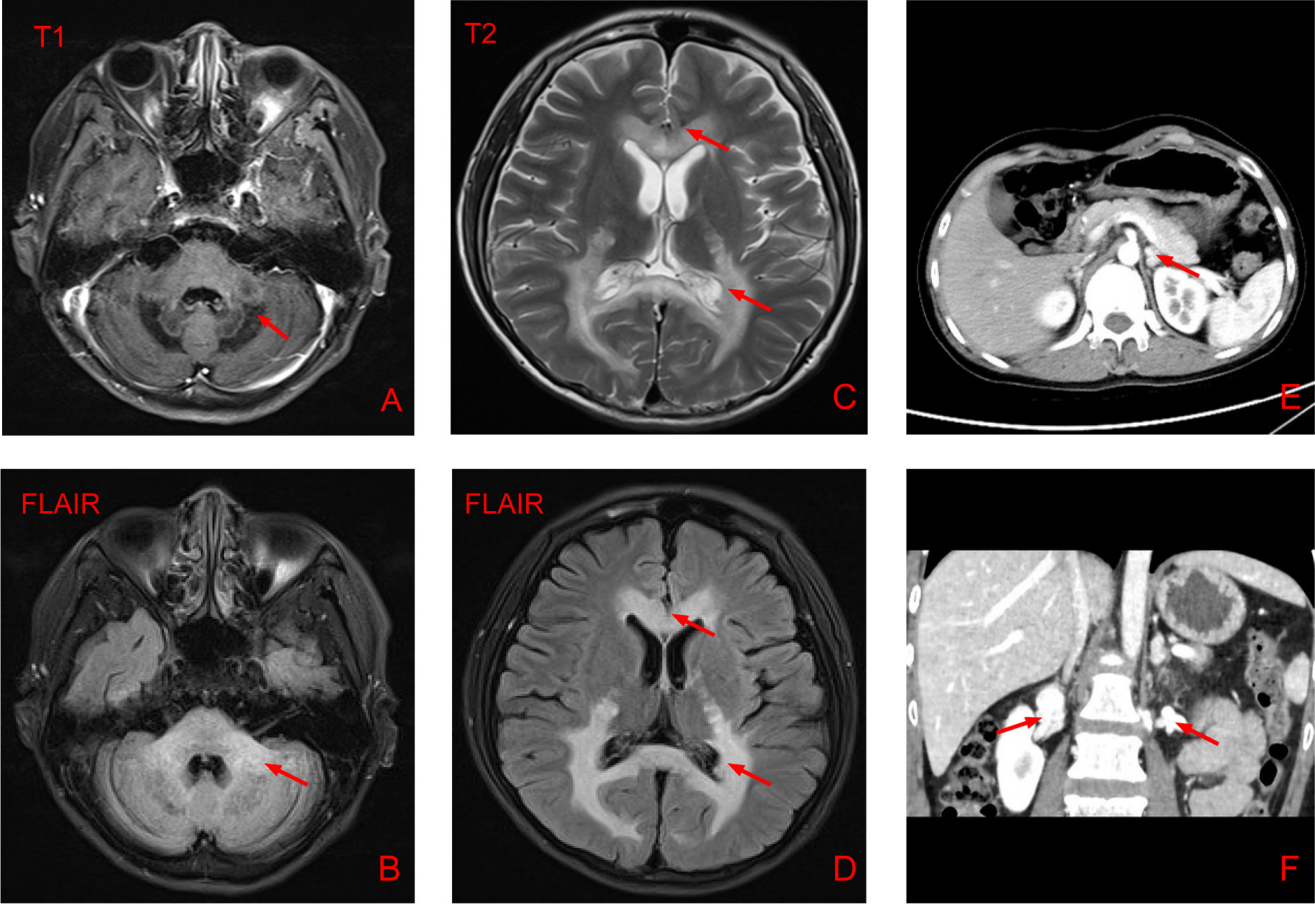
Results of brain magnetic resonance imaging and abdominal computed tomography (A-D) Brain MRI of the patient showing multiple patchy symmetrical subtle T2-weighted high-signal-intensity, T1-weighted low-signal-intensity and T2-FLAIR-high-signal-intensity lesions in the bilateral white matter around the lateral ventricle, posterior limbs of the internal capsule, and genu and splenium of the corpus callosum, brainstem and cerebellum. Mild enlargement of the bilateral third ventricle, fourth ventricle and lateral ventricle was observed. (E-F) Abdominal CT showing nodular hyperplasia in the bilateral adrenal glands and calcification in the left gland.
